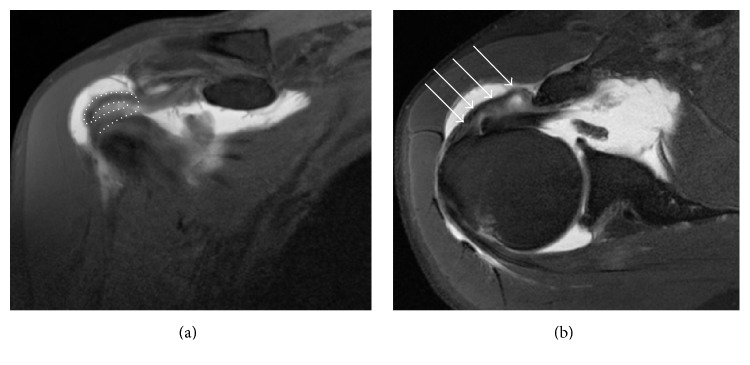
Coronal T1-weighted (a) and axial T2-weighted (b) with fat saturation of a right shoulder. The supraspinatus flopped on itself (white dotted line) and healed on the anterior humerus (white arrows).
